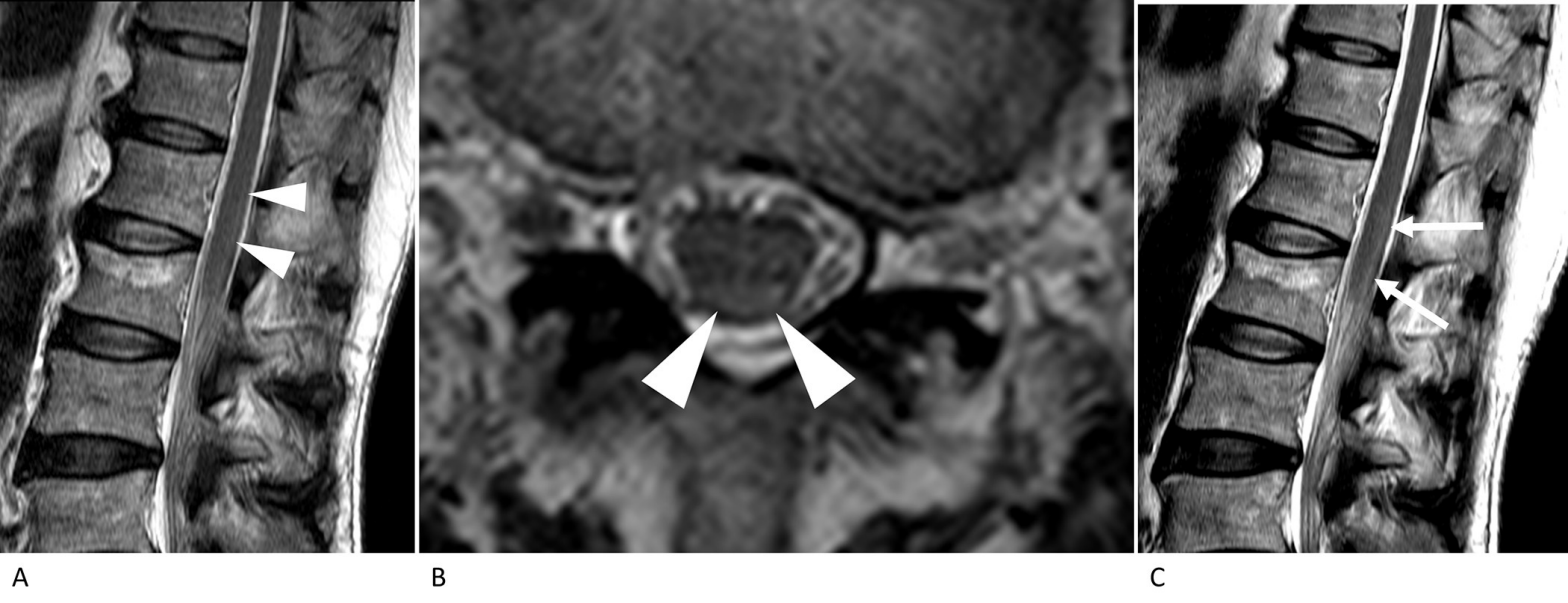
T2-weighted MRI of the thoracolumbar spine in the patient with spinal cord infarction (76-year-old man, patient number 1 in Table 4). On the sagittal (A) and axial (B) images obtained between 12 and 24 h after symptom onset, mild hyperintensity is suspected at the dorsal spinal cord (arrowheads). The authors evaluated the confidence score to be 3. Follow-up MRI (C) performed between 24 and 72 h after symptom onset shows pencil-like hyperintensity at the dorsal aspect of the spinal cord (arrows). The authors evaluated the confidence score to be 4.
